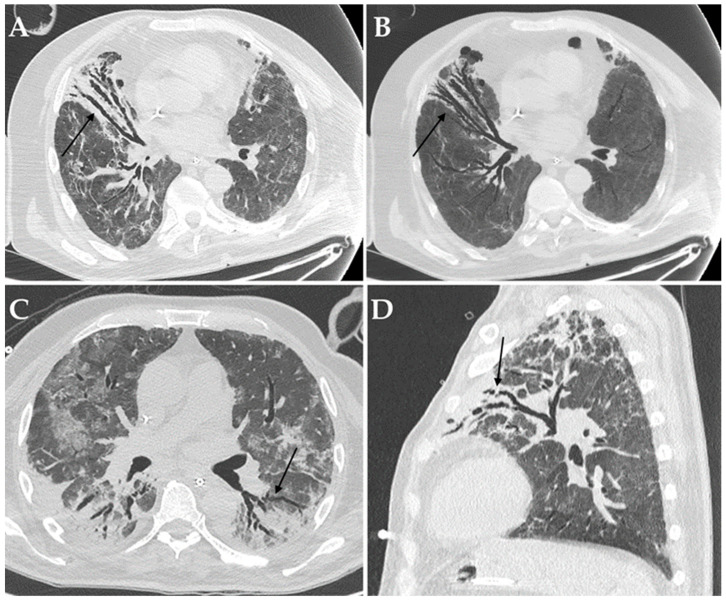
Axial HRCT images of a patient with COVID-19 ARDS admitted to ICU with bronchiectasis in the middle lobe (black arrow in (A)), being more evident at Minimum Intensity Projection (MIP) reconstruction (black arrow in (B)); *Aspergillus niger* was later detected in the bronchoalveolar lavage of the patient. Axial HRCT image of a different patient with COVID-19 ARDS with superinfection by *Klebsiella pneumoniae* and bronchiectasis in the dorsal segment of the left upper lobe (black arrow in (C)). Sagittal HRCT image of a different patient with COVID-19 ARDS showing bronchiectasis in the left upper lobe (black arrow in (D)) and presenting with elevated serum-galactomannan levels compatible with *Aspergillus* spp. superinfection.
